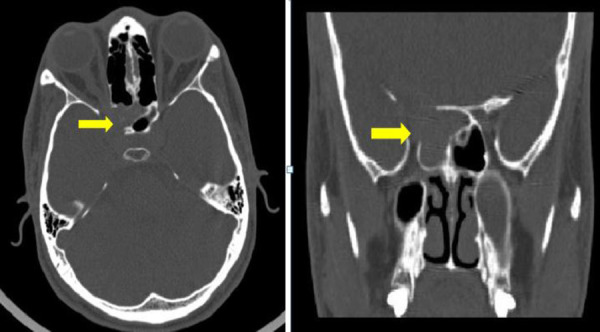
CT axial and coronal images showing the soft tissue with hyper dense foci seen in right sphenoid sinus with erosion of lateral wall and involving orbital apex.
